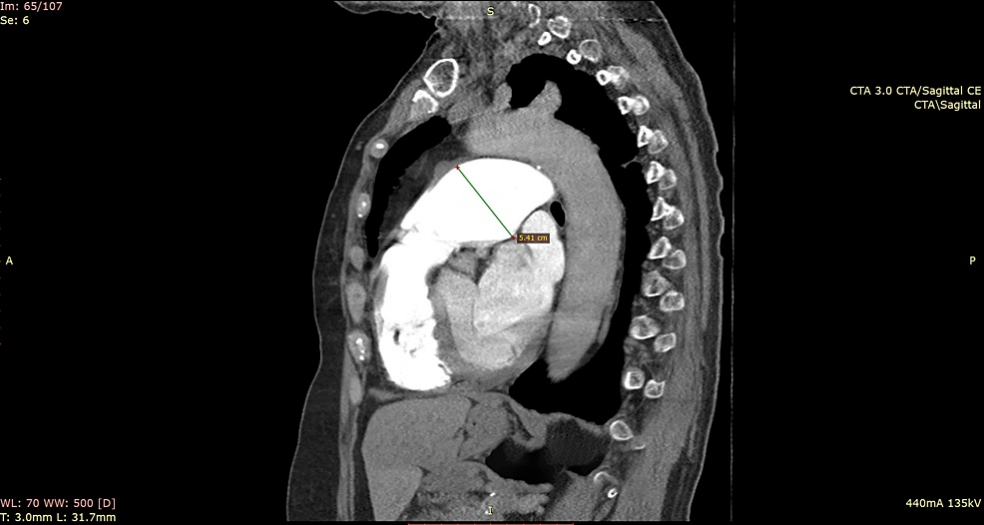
Preoperative computed tomography pulmonary angiogram from October 2020 The angiogram is showing a pulmonary artery aneurysm of 5.4 cm.
